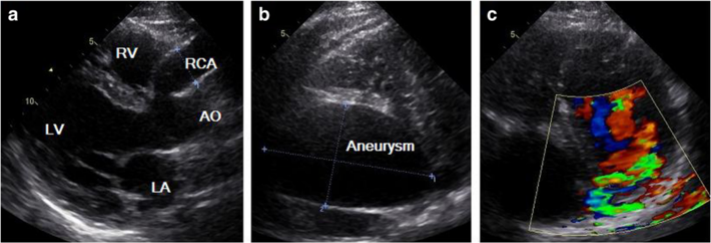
Transthoracic echocardiography (a) The obviously dilated RCA, the diameter of which was 2.2 cm. b The giant aneurysm measuring 10.0 × 6.1 cm. c The fistula draining into the LV through the posterior annulus of the mitral valve
